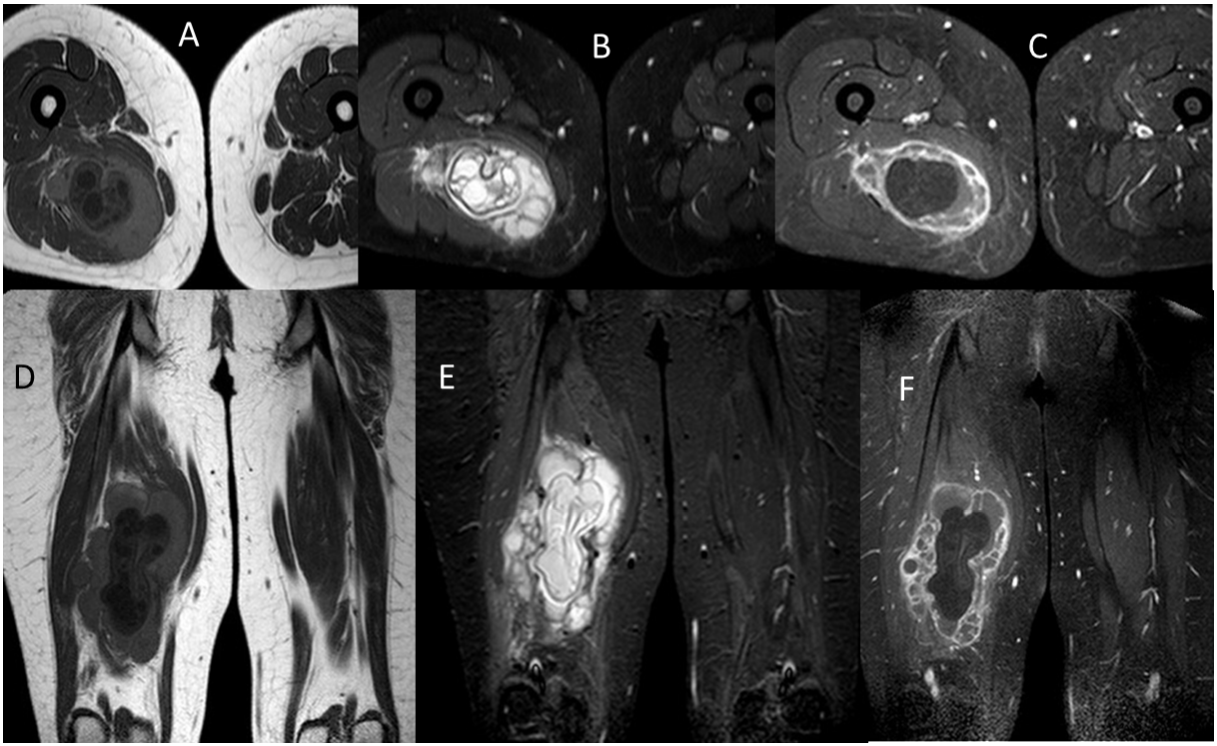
Post-contrast-enhanced images obtained by magnetic resonance imaging. Axial T1A (A), axial T2A (B), post-contrast-enhanced axial T1A (C), coronal T1A (D), coronal T2A (E), post-contrast-enhanced coronal T1A (F) images showing a lesion compatible with a hydatid cyst in the adductor brevis muscle.
